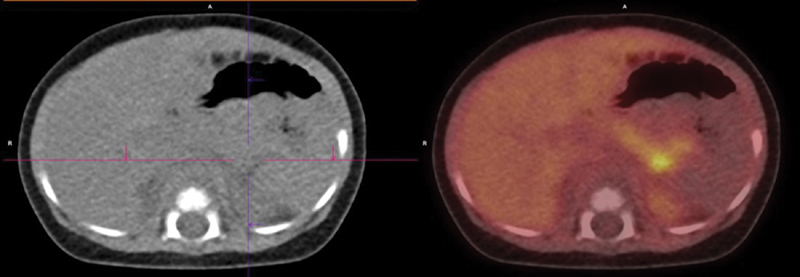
Abdominal 18F-fluoro-L-dihydroxyphenylalanine-positron emission tomography/computed tomography scan showing a hypermetabolic nodule in the distal portion of the body of the pancreas.
